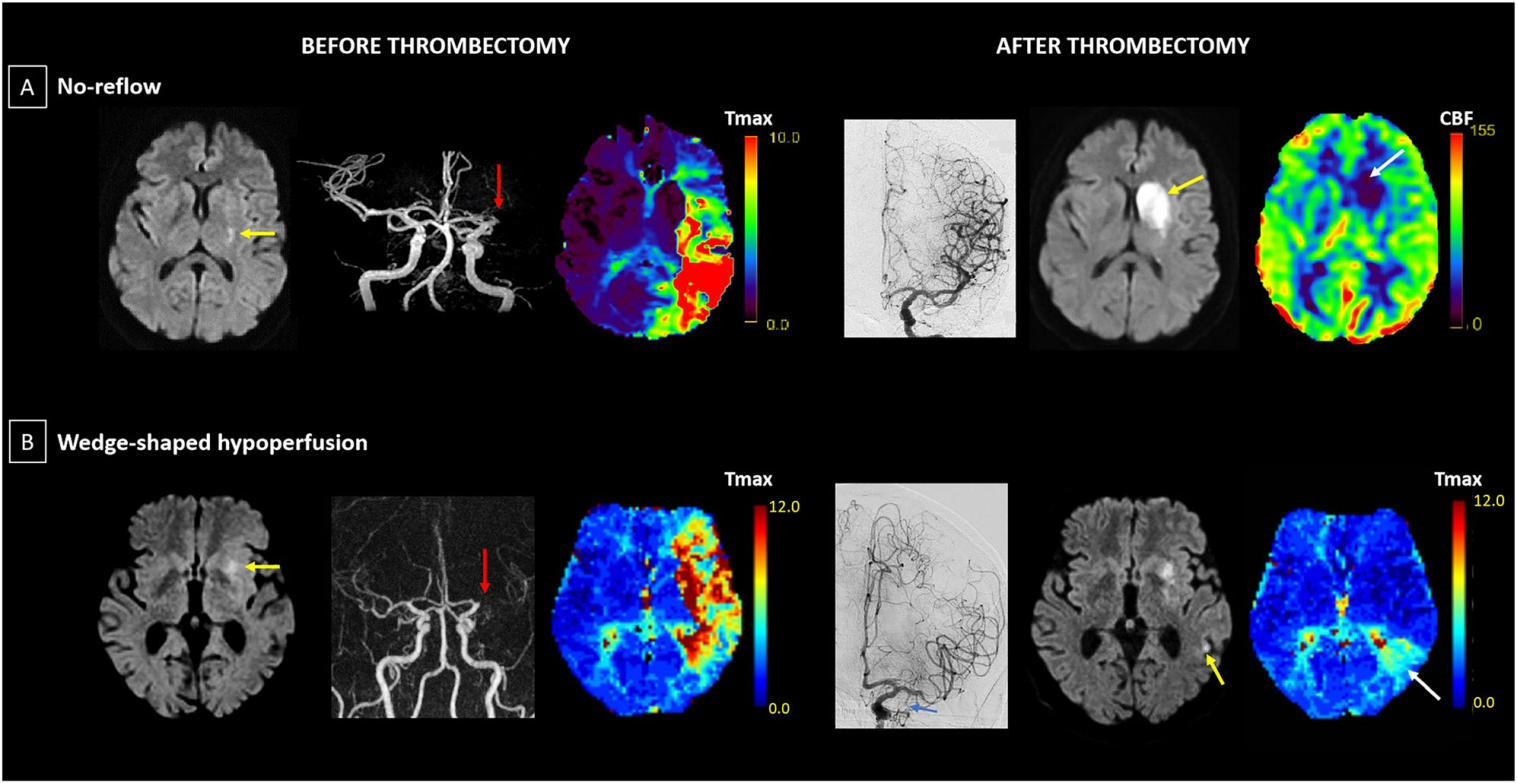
No‐reflow versus wedge‐shaped hypoperfusion after successful thrombectomy. Panel (A) “No‐reflow” pattern (Patient 1; adapted from [ter Schiphorst et al]^56^). The patient was admitted for hemiparesis and global aphasia with partial improvement on arrival resulting in an NIHSS of 4. Left‐to‐right, images acquired before thrombectomy (MRI, obtained 84 minutes after symptom onset): (1) DWI showing the baseline ischemic core (*yellow arrow*); (2) time‐of‐flight angiography demonstrating an M1 occlusion (*red arrow*); and (3) Tmax perfusion maps (in seconds) with a large delay in the corresponding territory. The patient was treated by thrombectomy 160 minutes after symptom onset. Left‐to‐right, images acquired after thrombectomy: (4) final DSA documenting successful recanalization (mTICI 3); (5) DWI at approximately 24 hours showing infarct extension to the entire caudate and lentiform nuclei (*yellow arrow*); and (6) ASL‐CBF (in ml/100 g/min) at approximately 24 hours revealing “no‐reflow” in the newly infarcted territory (*white arrow*). There was no evidence of re‐occlusion or hemorrhagic transformation on the approximately 24 hour MRI (MRA and T2*; not shown). Panel (B) “Wedge‐shaped hypoperfusion” pattern (Patient 2; in‐house case). The patient was admitted for facial paralysis and dysarthria, with an initial NIHSS score of 2. Left‐to‐right, images acquired before thrombectomy (MRI, obtained 95 minutes after symptom onset): (1) DWI showing the baseline ischemic core (*yellow arrow*); (2) contrast‐enhanced MRA (gadolinium) confirming an M1 occlusion (*red arrow*); and (3) Tmax perfusion maps (in seconds) with a territory‐concordant perfusion delay. The patient was initially treated with intravenous thrombolysis only, administered 120 minutes after symptom onset. Due to neurological deterioration leading to an NIHSS score of 6, a thrombectomy was subsequently performed 300 minutes after symptom onset. Left‐to‐right, images acquired after thrombectomy: (4) final DSA documenting an mTICI2b recanalization with persistence of an inferior branch occlusion (*blue arrow*); (5) DWI at approximately 24 hours showing an infarct growth (*yellow arrow*); (6) Tmax perfusion maps (in seconds) at approximately 24 hours showing a wedge‐shaped hypoperfusion in the same territory (*white arrow*), consistent with a distal embolus, which was visible in the concomitant time‐of‐flight imaging (not shown). ASL = arterial spin labeling; CBF = cerebral blood flow; DWI = diffusion‐weighted imaging; DSA = digital subtraction angiography; M1 = first segment of the middle cerebral artery; MRA = magnetic resonance angiography; MRI = magnetic resonance imaging; mTICI = modified treatment in cerebral infarction; NIHSS = National Institutes of Health Stroke Scale; Tmax = time to maximum concentration. [Color figure can be viewed at www.annalsofneurology.org]
